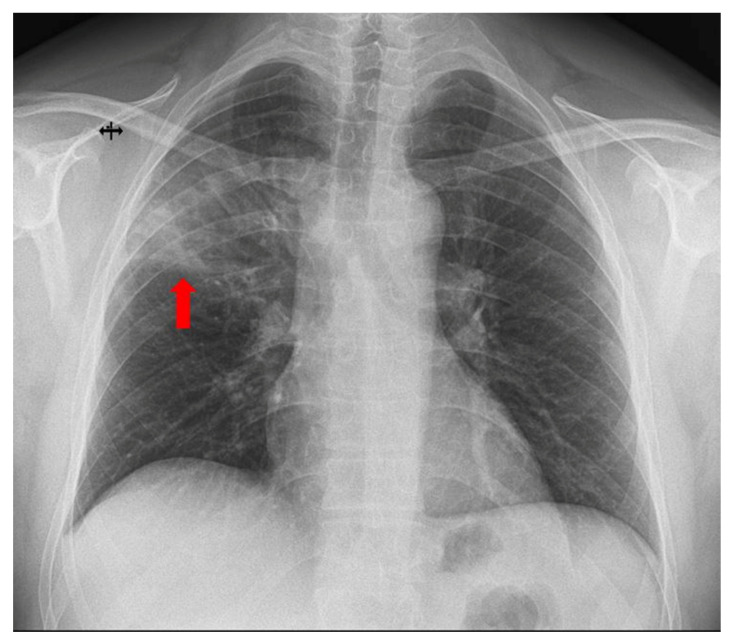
A 42-year-old non-smoking male electrician with occupational exposure to respiratory irritants presented to the emergency department with a 24 h history of fever (38.7 °C), non-productive, irritating cough, and diffuse post-tussive chest pain. His past medical history included SARS-CoV-2 infection four years earlier and mixed dyslipidemia controlled with statins and fenofibrate. On admission, he was febrile (38.7 °C), normotensive, and maintained normal oxygen saturation. Lung auscultation revealed bilateral vesicular breath sounds without additional adventitious sounds. Laboratory evaluation demonstrated an inflammatory response (elevated C-reactive protein—108 mg/L (0–5 mg/L) and procalcitonin—0.17 ng/mL (0–0.05 ng/mL)), with normal value of leukocytes (WBC—9.99 × 10^3^/µL, neutrophils—6.86 × 103/µL, lymphocytes—1.82 × 10^3^/µL), and hypertriglyceridemia. Chest X-ray revealed a subpleural, pseudo-triangular opacity of inhomogeneous density in the upper third of the right lung field (red arrow). For further evaluation of the radiographic abnormality and pulmonary function, spirometry with bronchodilator testing and thoracic CT were recommended.
